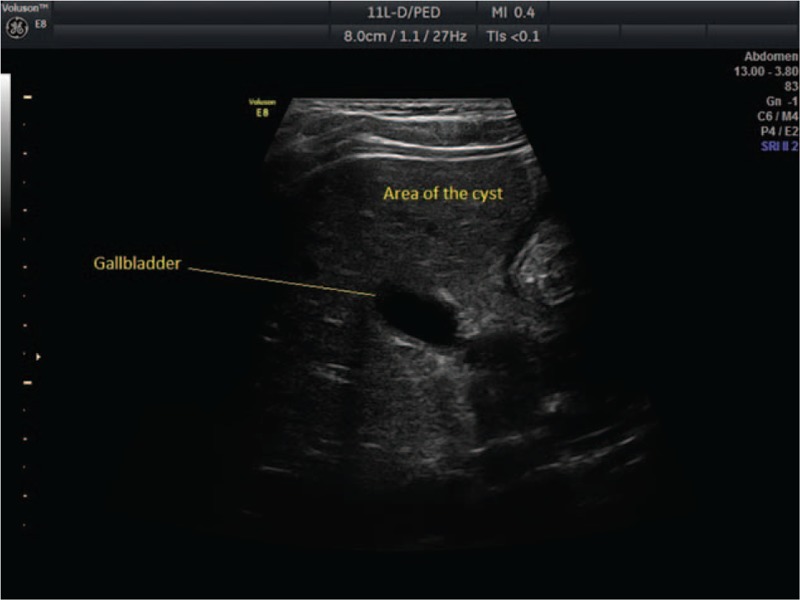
Transabdominal ultrasound showing resolution of liver cyst at 18 months of age.
